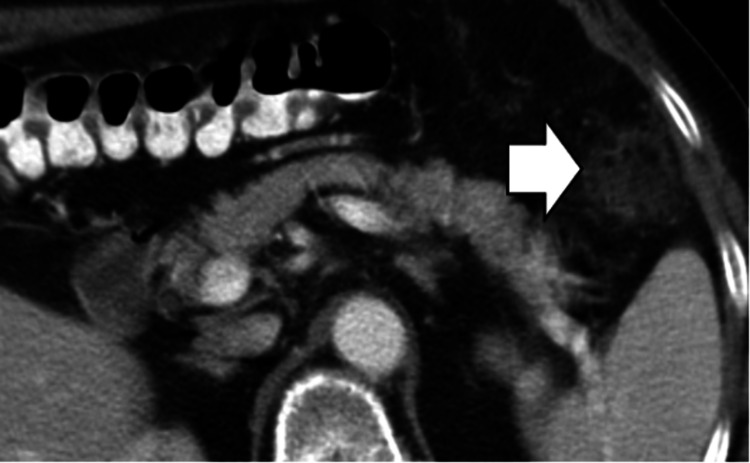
Omental infarct. CT image shows localized soft tissue stranding in the left upper quadrant omentum (white arrow).
